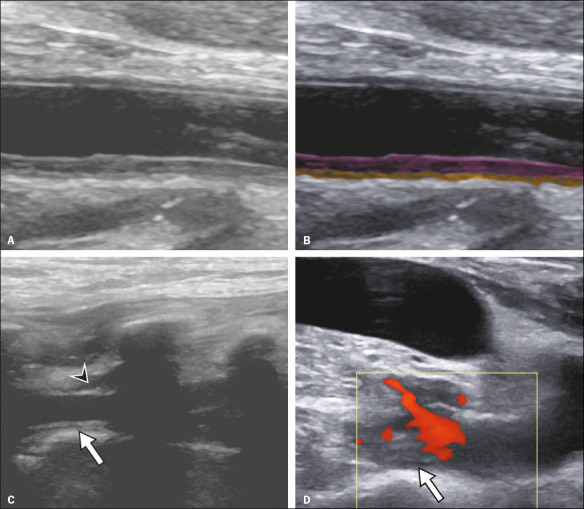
A 50-year-old woman with COVID-19. Grayscale ultrasound image
